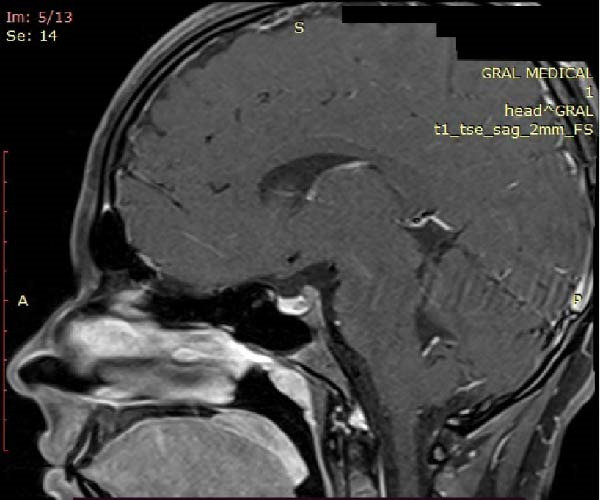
Sagittal T1‐weighted fat‐suppressed post‐contrast MRI showing a well‐defined nonenhancing lesion within the anterior pituitary gland, surrounded by enhancing tissue.
